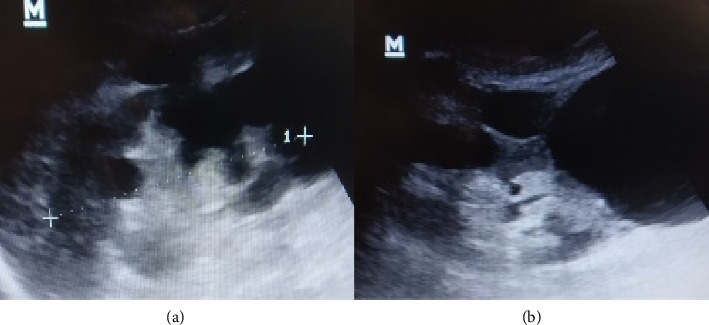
Kidneys ultrasound; showed multiple and diffuse cysts in both left (a) and right (b) kidneys.
